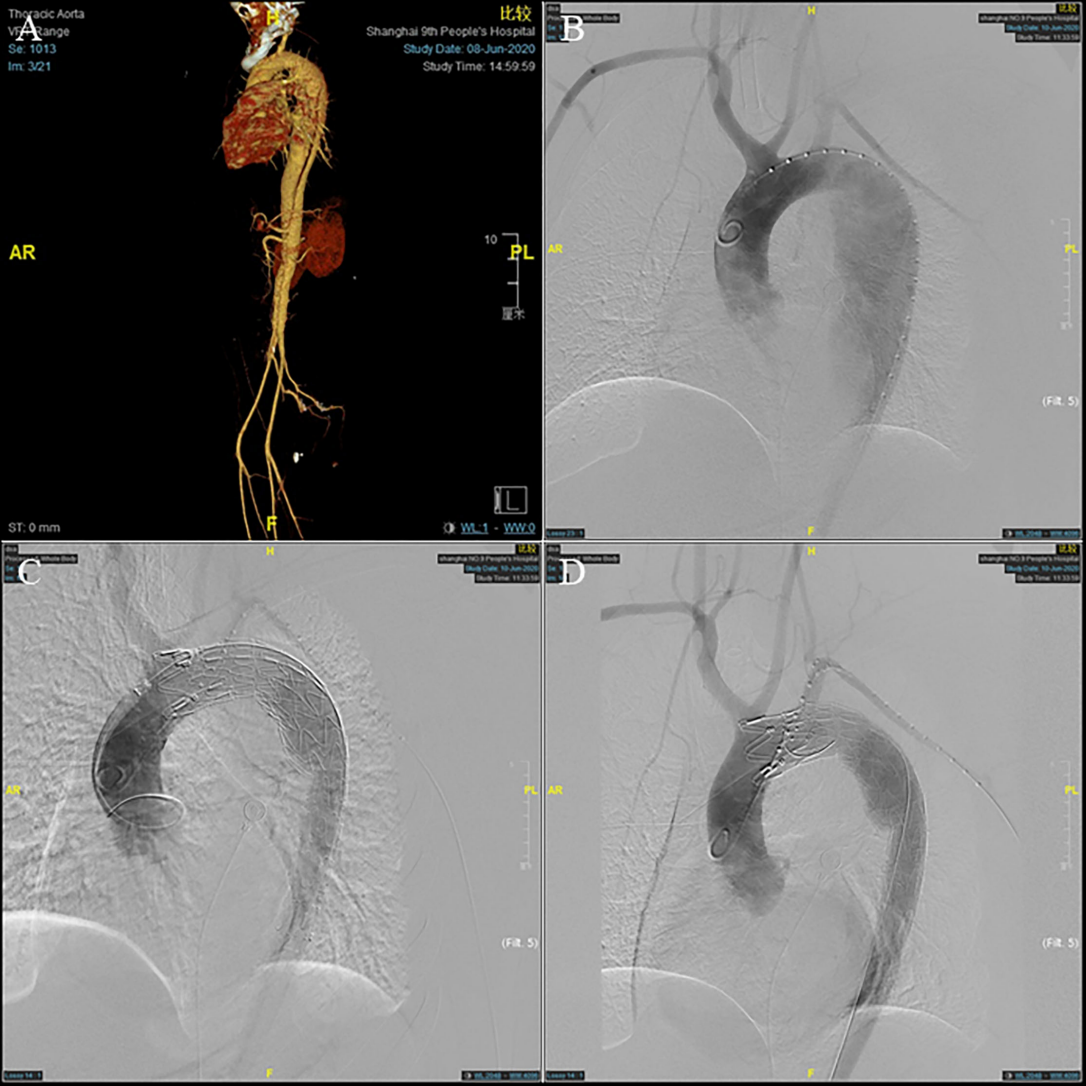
A 45-year-old man who presented with 7 days of chest and back pain was diagnosed as type B aortic dissection (TBAD). (A) Pre-operative computed tomographic angiography (CTA) and (B) intra-operative angiography showed a pathologically changed aorta arch. (C) The stent graft was deployed to seal the aortic arch lesion, leaving its distal end partially within the restrictive covered stent (RCS). (D) After left subclavian artery (LSA) fenestration, post-operative angiography showing satisfactory repair of the dissected aorta.
